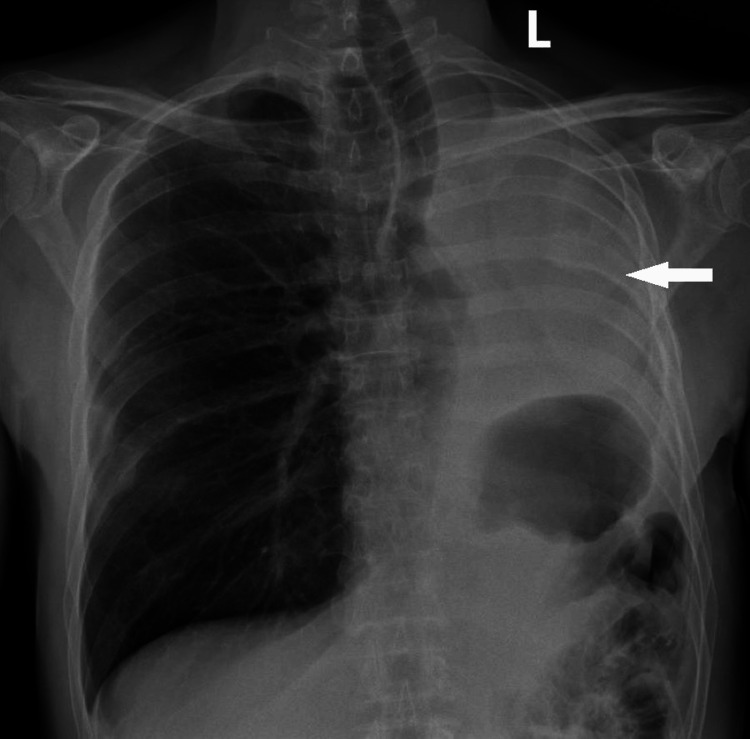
Five-month follow-up chest X-ray showing left side mediastinal shift with fibrinoid collection in the pneumonectomy cavity.
